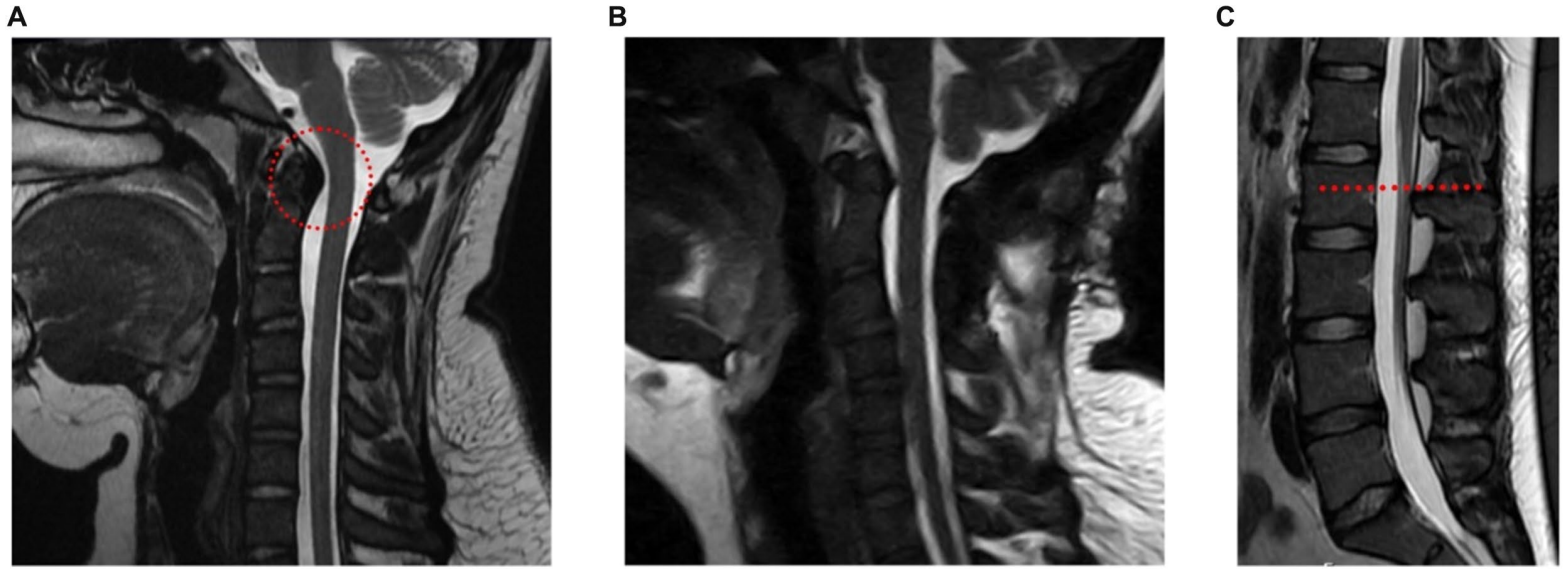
A young woman with hEDS, CCI, and TCS. (A) Upright cervical flexion MRI showing medullary kinking (red circle) (clivo-axial angle of 116 degrees). (B) Postoperative upright MRI showing reduced medullary kinking after fixation and fusion. (C) MRI of lumbar spine showing a slightly low-lying conus at mid L2 body (red line).
